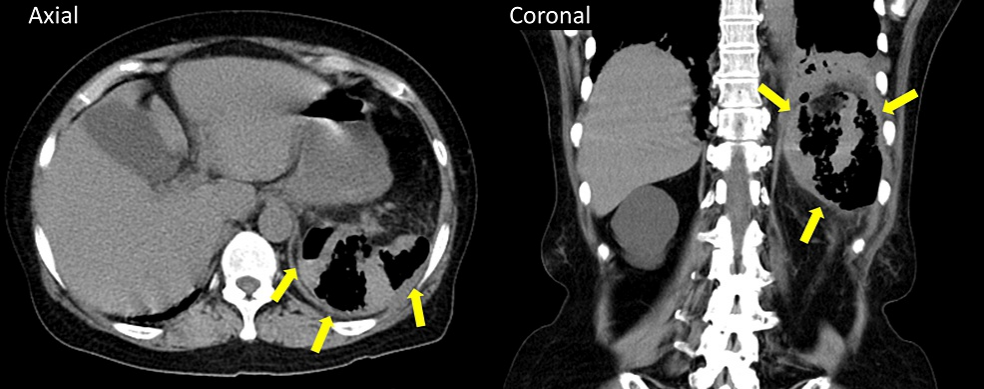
The axial and coronal planes of the CT show gas gangrene of the spleen Yellow arrows indicate gas in the spleen.
